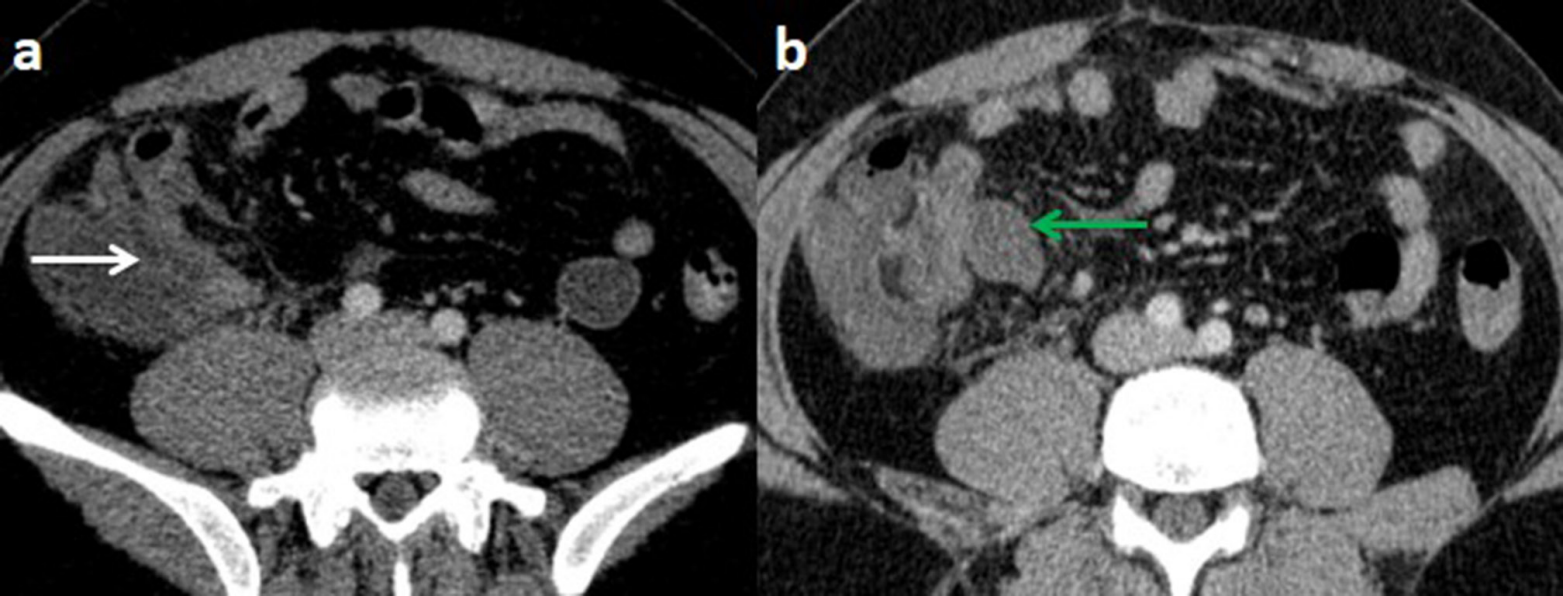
Computed tomography scan of the abdomen with intravenous contrast at portal phase (with no oral contrast) on axial plan (a,b) showing an enlarged and thick-walled appendix (white arrow), with a fluid collection in the latero cecal region (green arrow).
